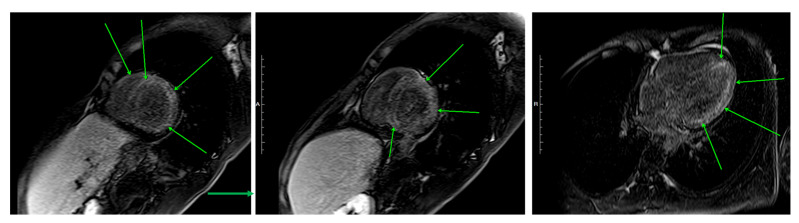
Cardiac MRI. Green arrows indicate areas of early gadolinium enhancement, reflecting myocardial injury.
